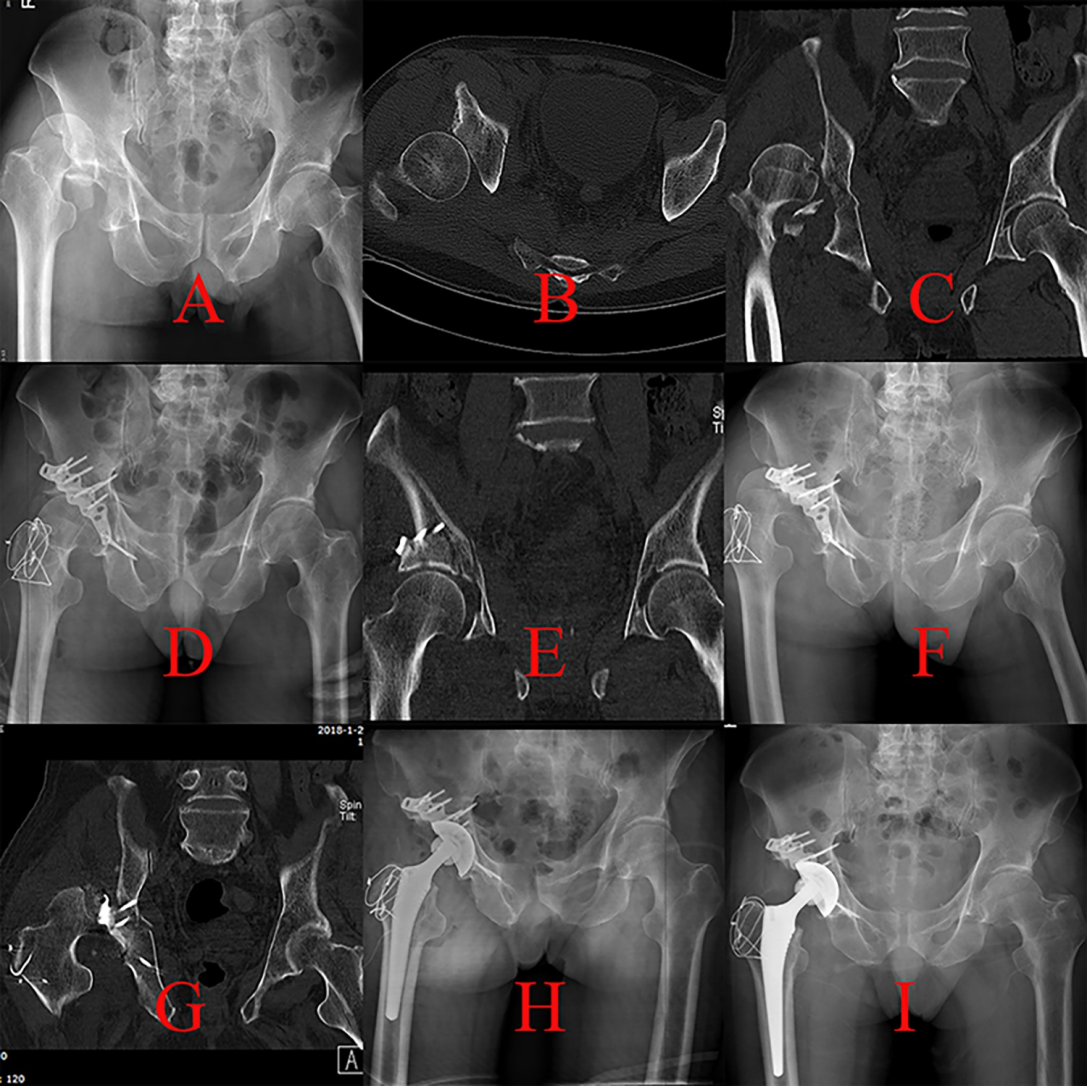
The therapeutic process of a patient with A3.1 injury who underwent the KL procedure was presented. A, cephalad displaced femoral head and displaced roof wall fragment (more cranial than traditional PW fracture) in an anteroposterior (AP) view; B-C, superior posterior dislocation of femoral head on CT scans; D, postoperative AP projection after the KL procedure; E, the femoral head was contained in a concentric acetabulum although there were residual displacements of roof wall fragments on postoperative CT; F, secondary dislocation was noted 3 months postoperatively; G, dislocation and necrosis of affected femoral head on a CT scan; H, AP view after THA surgery; I, AP view 6 months postoperatively.
